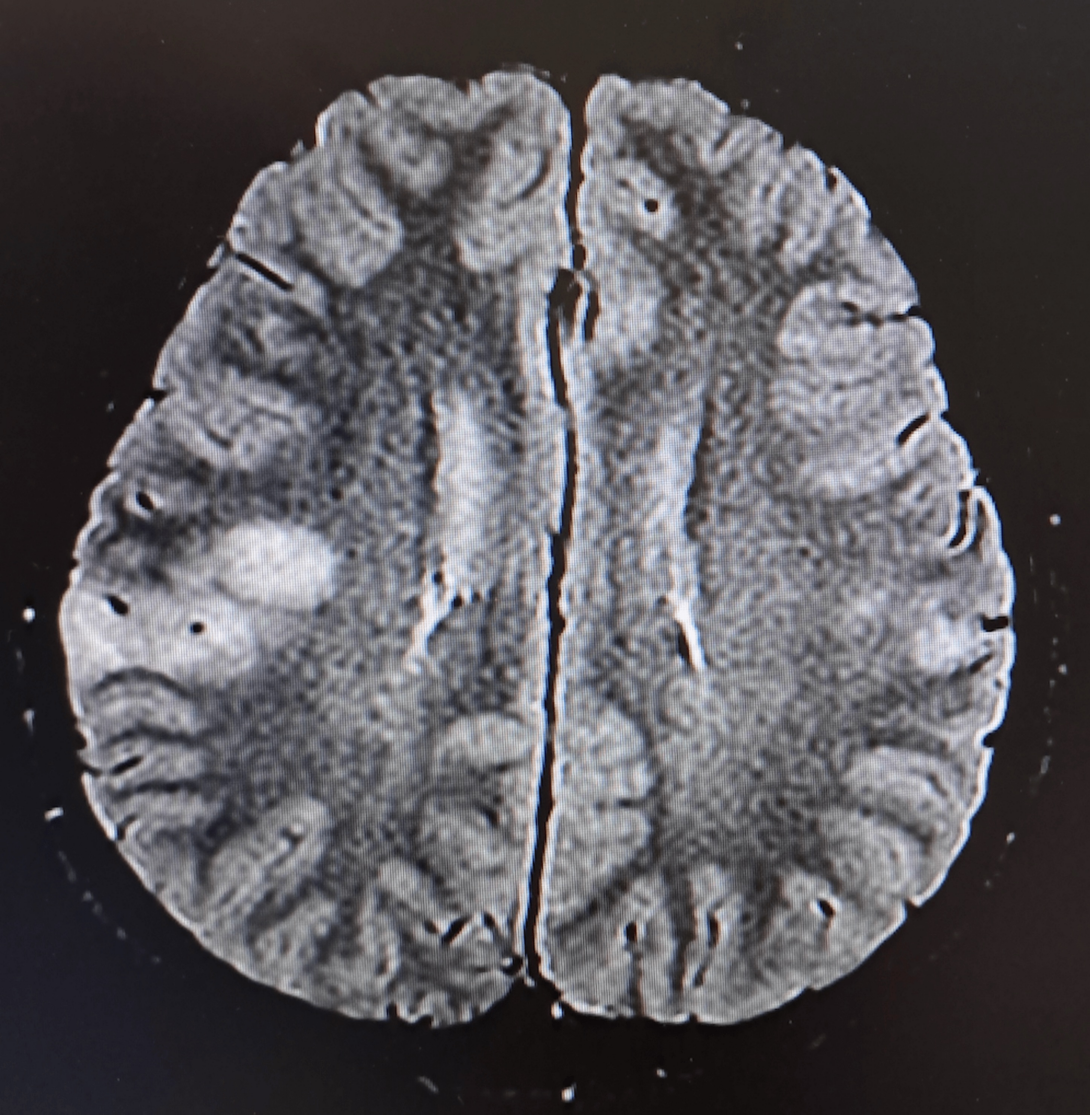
Axial T2 FLAIR MRI of the brain upon admission. It shows involvement of the right temporal and right high frontal gyri, with lesser involvement of the left high frontal gyrus.
